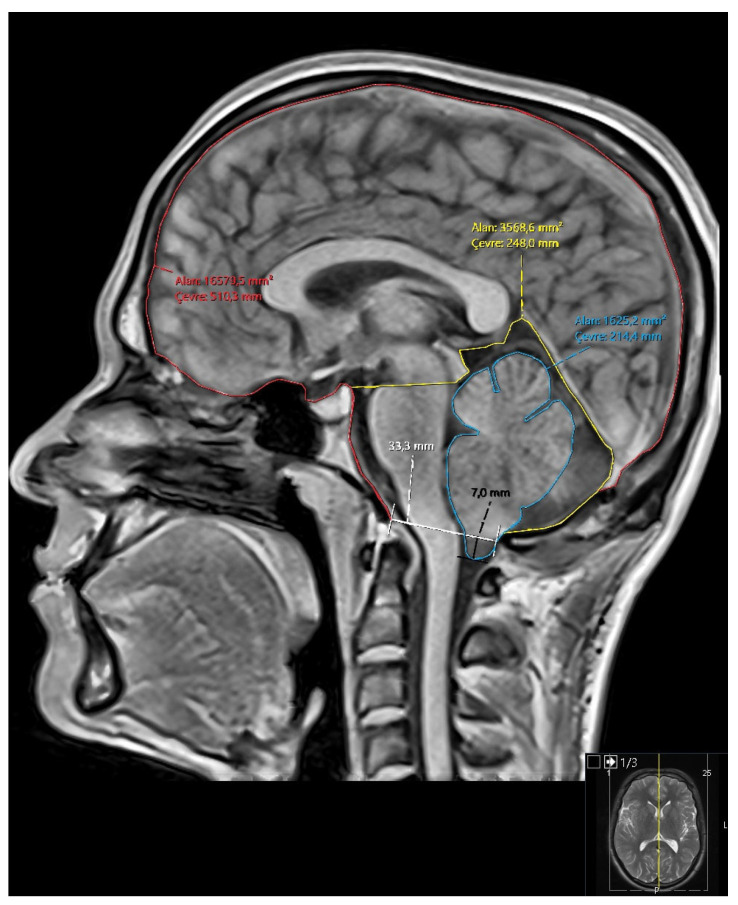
Representative mid-sagittal T1-weighted brain MRI demonstrating cranial morphometric measurements used in the study. Traced regions include the intracranial area (red), posterior fossa (yellow), and cerebellum (blue). Quantitative measurements include area and perimeter of each region, foramen magnum diameter (white), and degree of cerebellar tonsillar descent (black).
